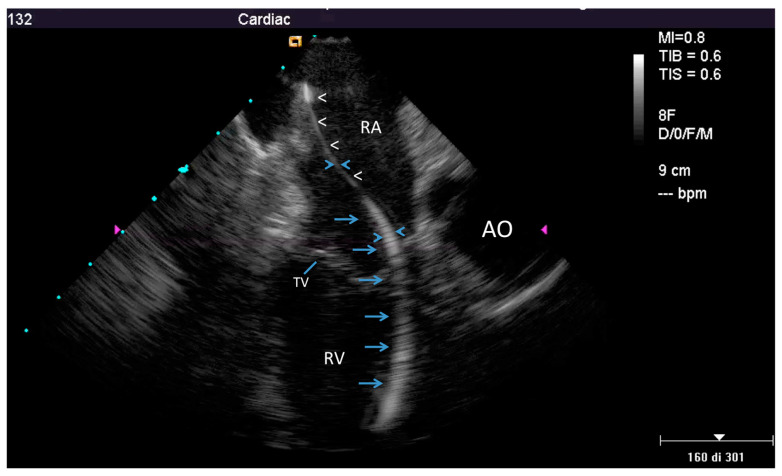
Example of a partially thickened lead at intracardiac echocardiography (ICE). Home view showing normal thickness of the proximal part of the lead in the right atrium (white arrow head), but evident thickening in the distal atrial part and in its ventricular course (≥4 mm, blue arrows); AO—aorta; RA—right atrium; RV—right ventricle; TV—tricuspid valve.
